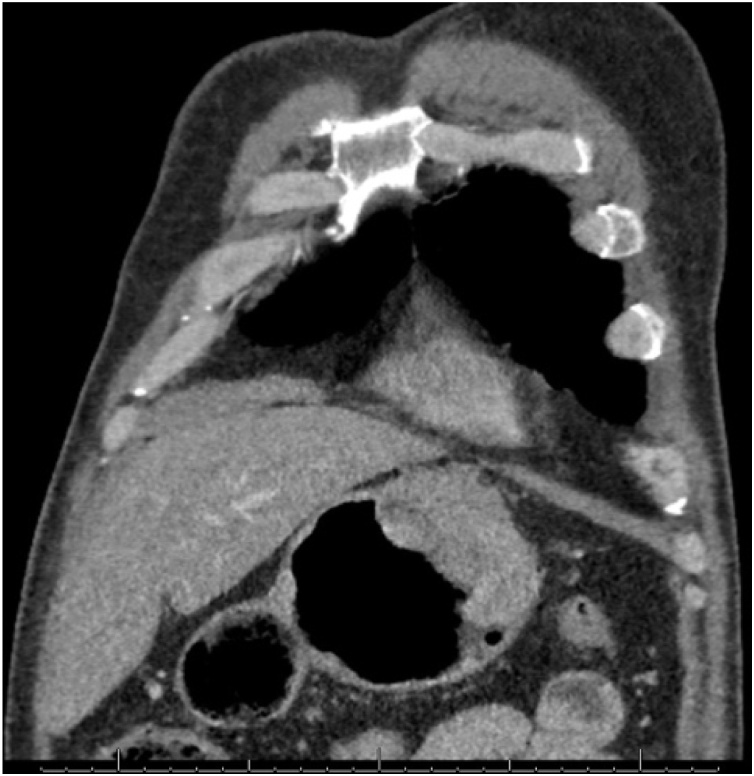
A coronal plane of CT abdomen showing large lobulated mass at the greater curvature.
